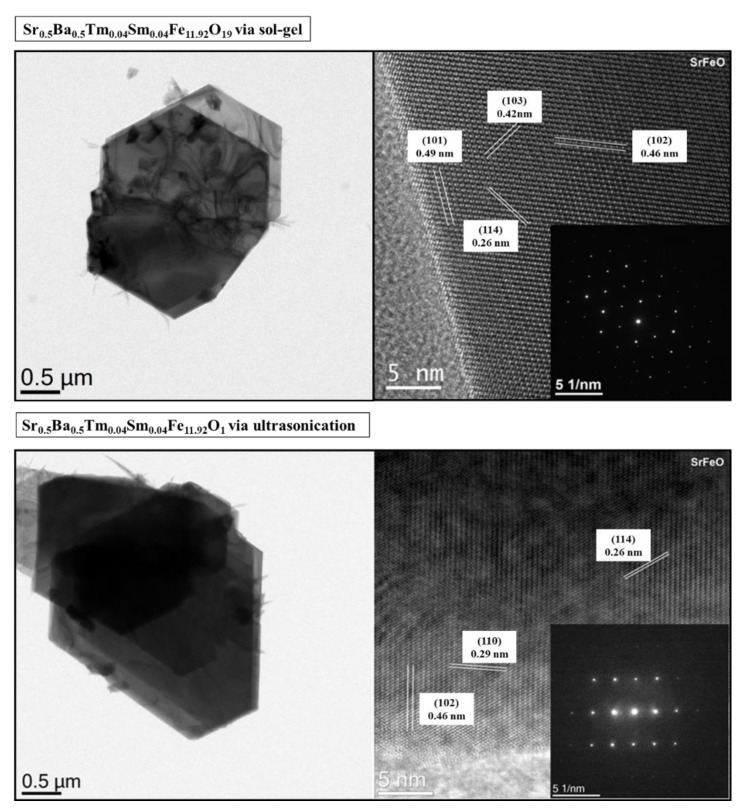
TEM and HR-TEM images of Sr_0.5_Ba_0.5_Tm*_x_*Sm*_x_*Fe_12−2*x*_O_19_ (*x* = 0.04) NHF prepared through both sol-gel auto-combustion and ultrasonication method.
